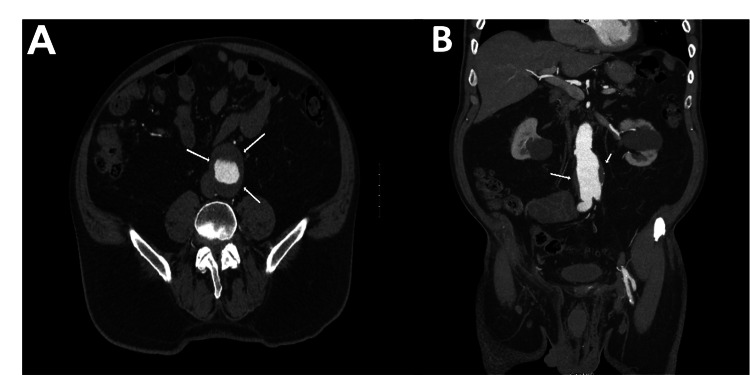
Abdominal aortic aneurysm: axial (panel A) and coronal (panel B) pre-procedural computed tomography angiograph slices showing the abdominal aortic aneurysm (white arrows).
